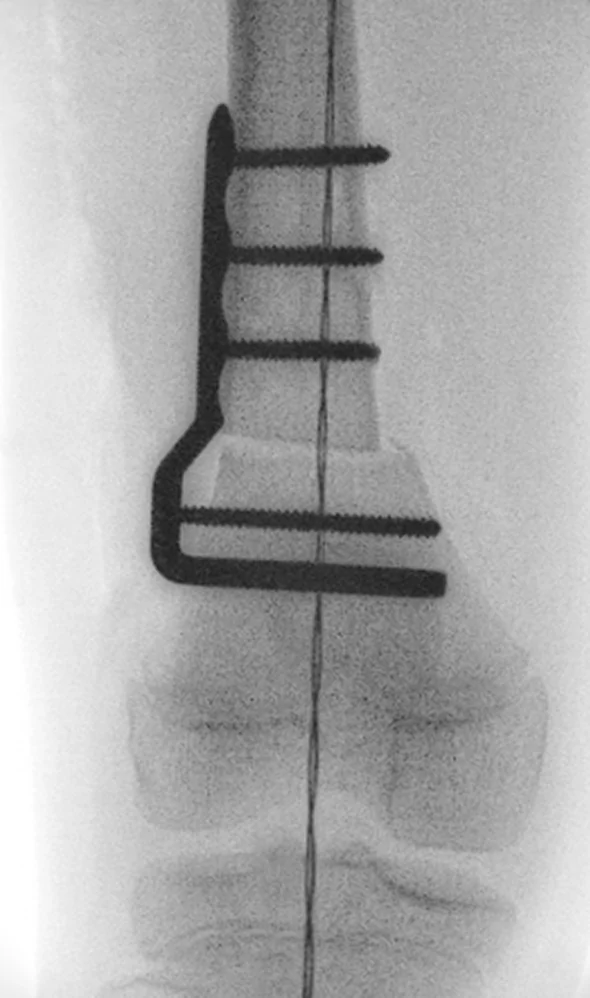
Final radiographic evaluation with projected leg axis
